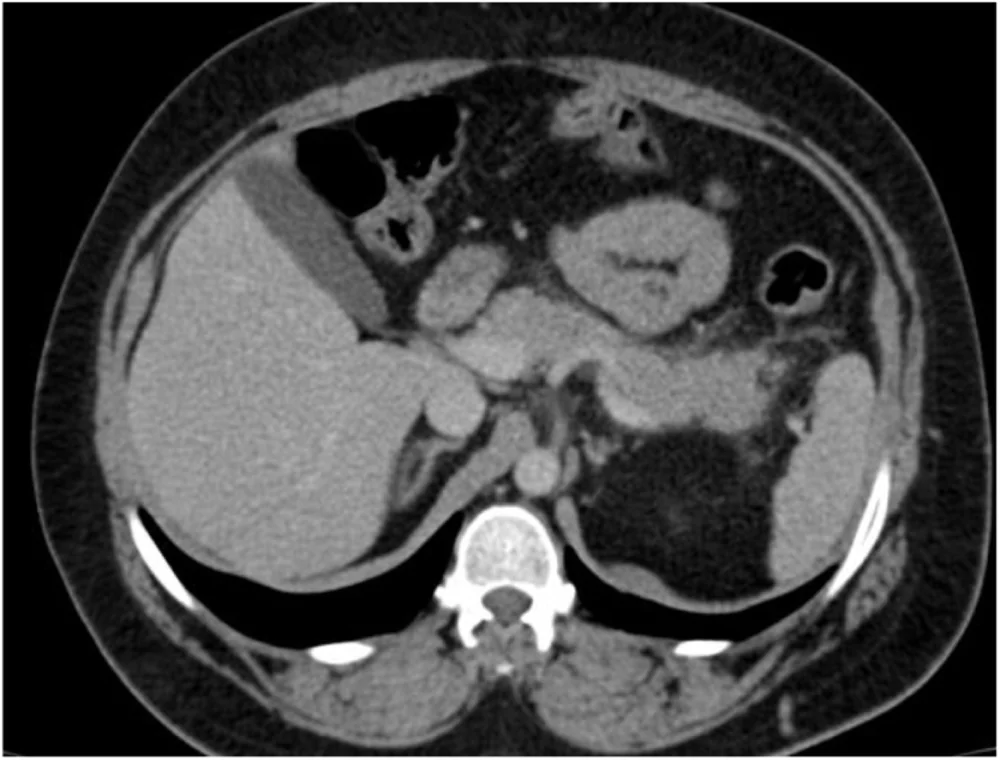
Computed tomography scan showing pancreatic edema.
